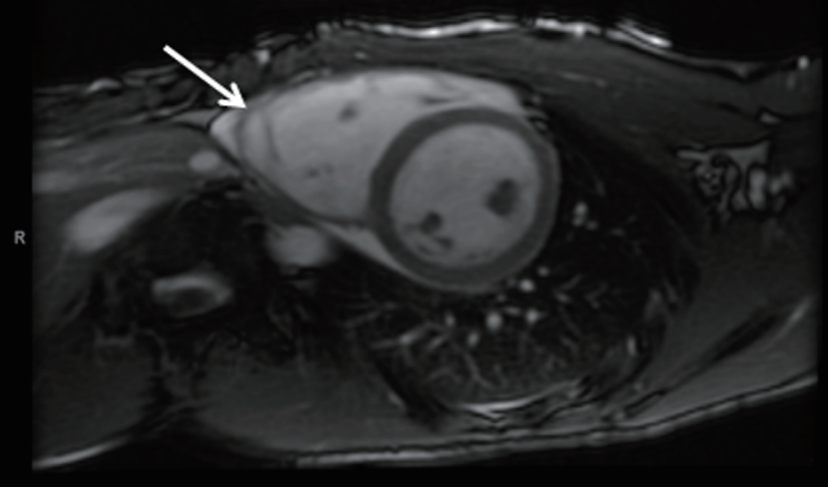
MRI in axial view shows the heart in diastole with a dilated right ventricle and trabeculation (arrow).
